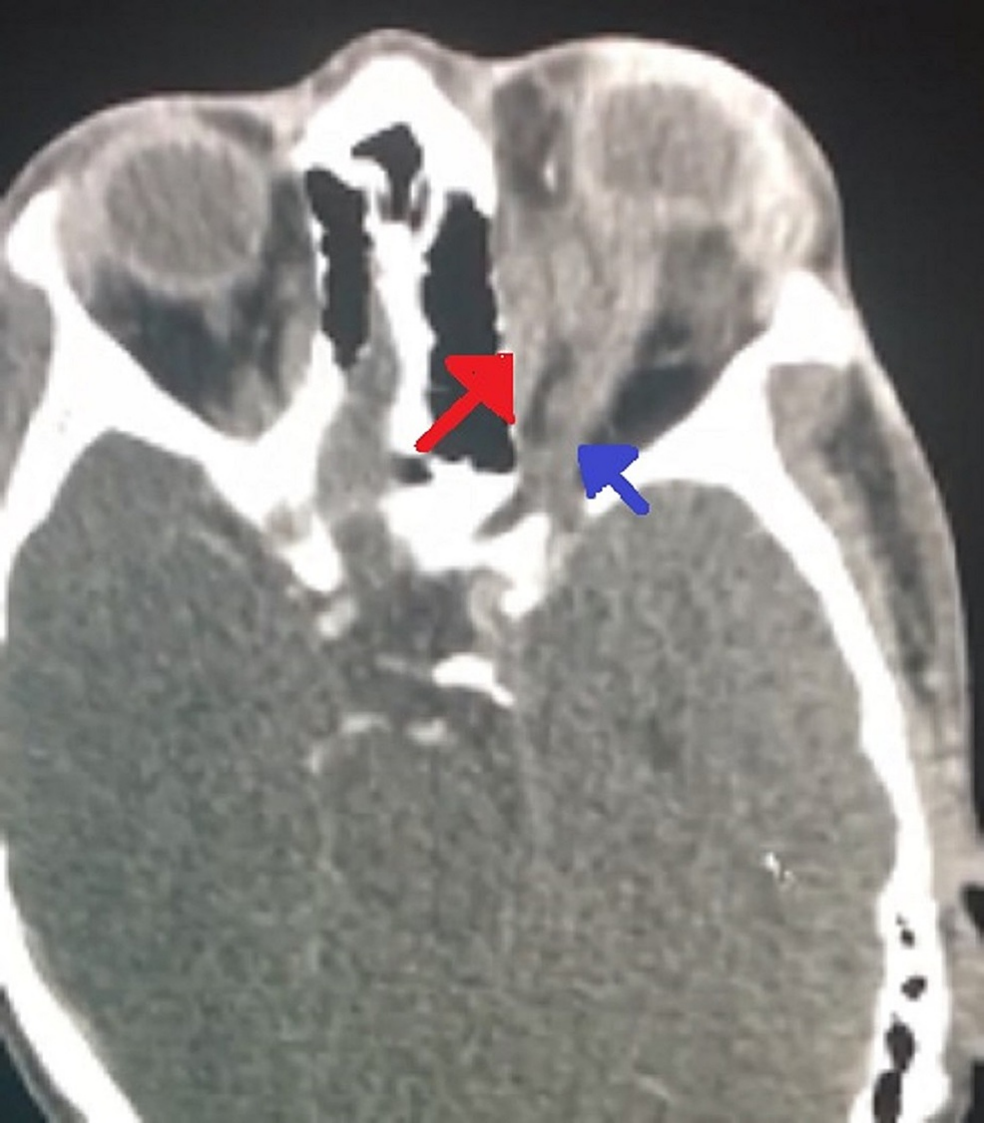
Axial computed tomography scan of both orbits showing left eye proptosis caused by nasal extraconal cystic mass (red arrow) and a kinked optic nerve (blue arrow).
